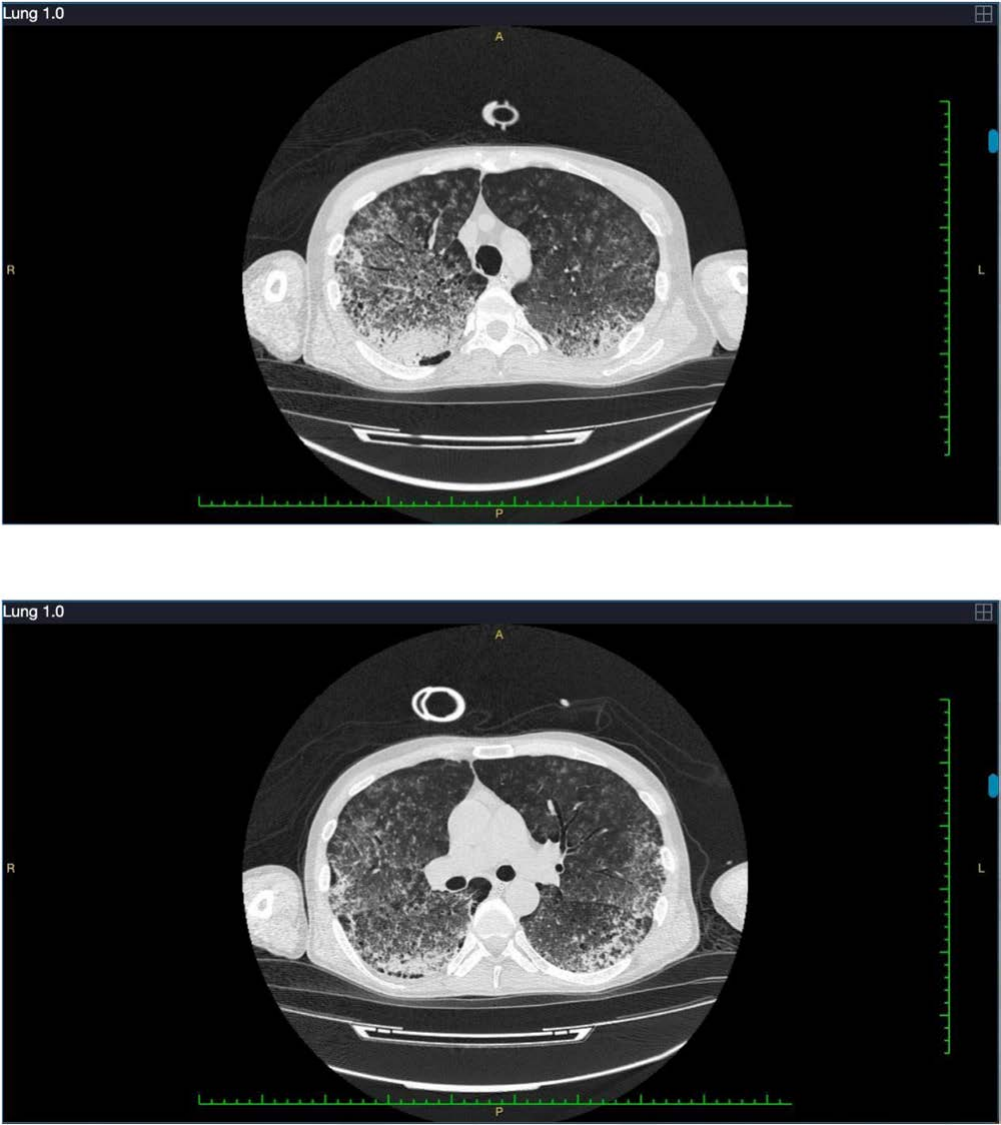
The chest computed tomography on the 9th day of hospitalization revealed patchy areas in both lungs.
